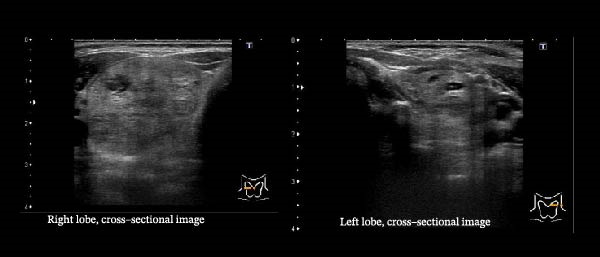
Thyroid ultrasound examination. Isthmus thickness: 8 mm, right lobe: 24 mm × 27 mm × 67 mm, and left lobe: 28 mm × 21 mm × 69 mm. Multiple adenomatous nodules occupied the entire lobe.
